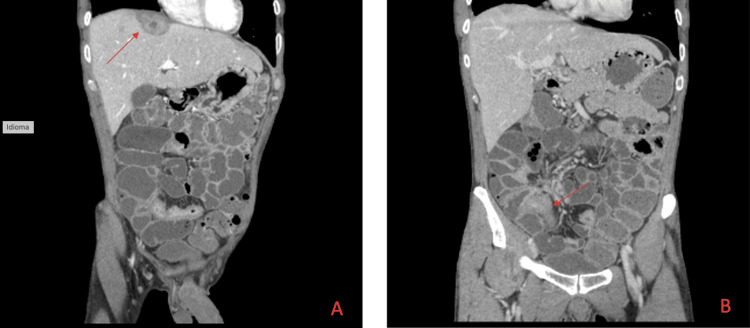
Abdominal Entero CT images of the pyogenic abscess and inflammation of the ileocecal valve. A: One pyogenic liver abscesses in the process of being resolved, identified by the red arrow. B: Diffuse segmental parietal enhancement of the terminal ileum, involving the ileocecal valve, identified by the red arrow.
